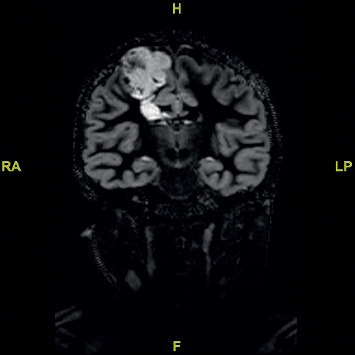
Coronal flair MRI demonstrating tumour in the primary motor cortex.
